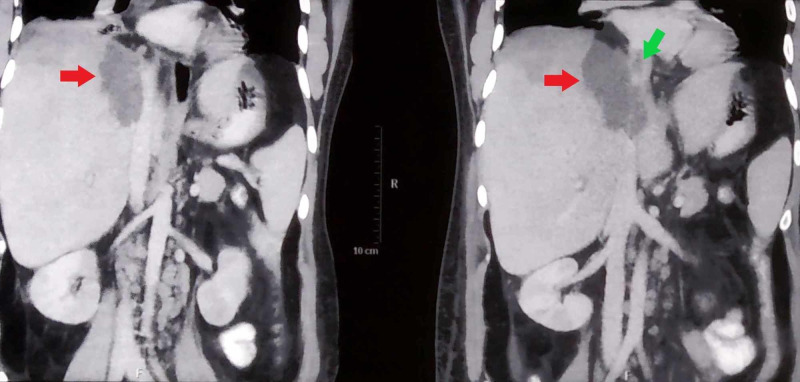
Coronal sections of computed tomographic (CT) scan of abdomen showing the liver abscess (red arrows) and thrombus in the adjacent inferior vena cava (green arrow).
